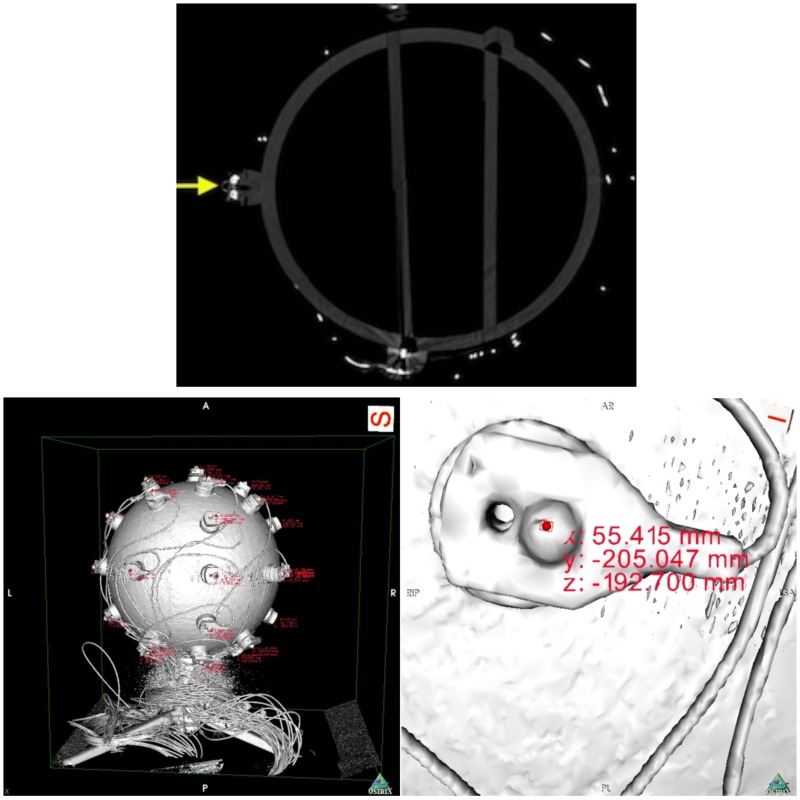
On top, a slice of the fiberglass sphere CT scan. The solid yellow arrow points at a sectioned electrode with a reflector on top. On the bottom, images of the sphere's reconstructed surface. On the left, and on the right a close up of an electrode. The pictures shows the reconstructed surface of the sphere and electrodes, obtained from the scan slices. The areas of red text are coordinates that are displayed when an investigator clicks on a part of the digitized model, in this case the top surface of the reflector markers. On the right, we can see the electrode with its reflector and corresponding displayed coordinates.
